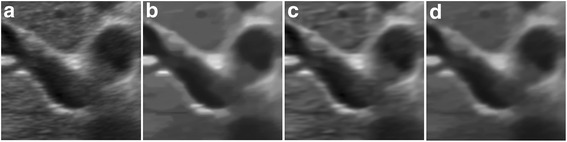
The representative regions corresponding to the red rectangle region in Fig. 6a for the liver ultrasound image. aThe original image. b-d Outputs of the compared filters, b BNLTV c SAR-BM3D, d The proposed method. BNLTV = Bayesian NLTV; SAR-BM3D = blocking matching 3D filter of SAR image
